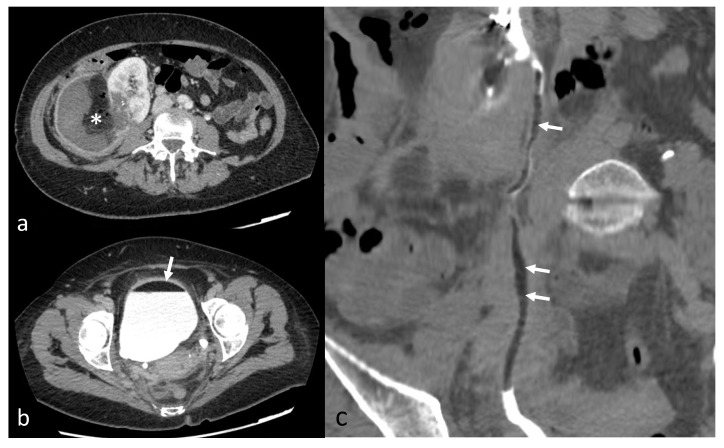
Postembolization lipuria in a 70-year-old patient with a right AML. (a) Intratumoral necrosis (*) on axial computed tomography with injection and urinary acquisition (b) Fat-supernatant indicating lipuria (white arrow) on axial computed tomography with injection and urinary acquisition (c) Intra-ureteral fat (white arrows) on coronal computed tomography with injection and urinary acquisition.
